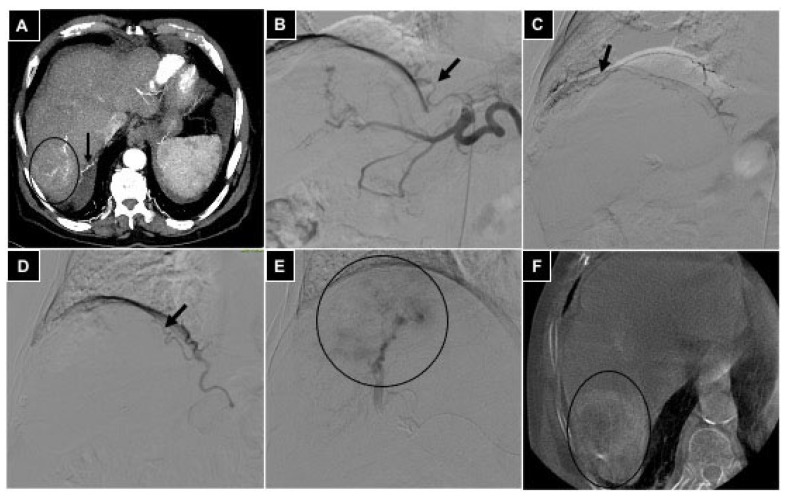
An 81-year-old male with a single 6 cm HCC nodule; treatment with TARE was selected. (A) A 6 cm HCC nodule in segment VII (black circle) is evident in the CT scan in the arterial phase; an additional lesion feeding from the right phrenic artery (black arrow) is appreciable. (B) During the preliminary work-up procedure, selective coeliac trunk arteriography is acquired via a 5 Fr diagnostic catheter positioned via femoral access, and a hypertrophic right phrenic artery is evident (black arrow). (C) A 2.4 Fr microcatheter is advanced into the right phrenic artery and a DSA is acquired, showing nodule refurnishment from small distal branches (black arrow). (D) Flow diversion by means of temporary embolization of the right phrenic artery (black arrow) is performed, and stop flow is obtained by Embocept-S^®^ microparticle injection. (E) The microcatheter is advanced into the right hepatic artery and a DSA is acquired, showing nodule refurnishment (black circle). (F) A ConeBeamCT is acquired before ^99m^Tc MAA injection and target opacification is evident (black circle).
